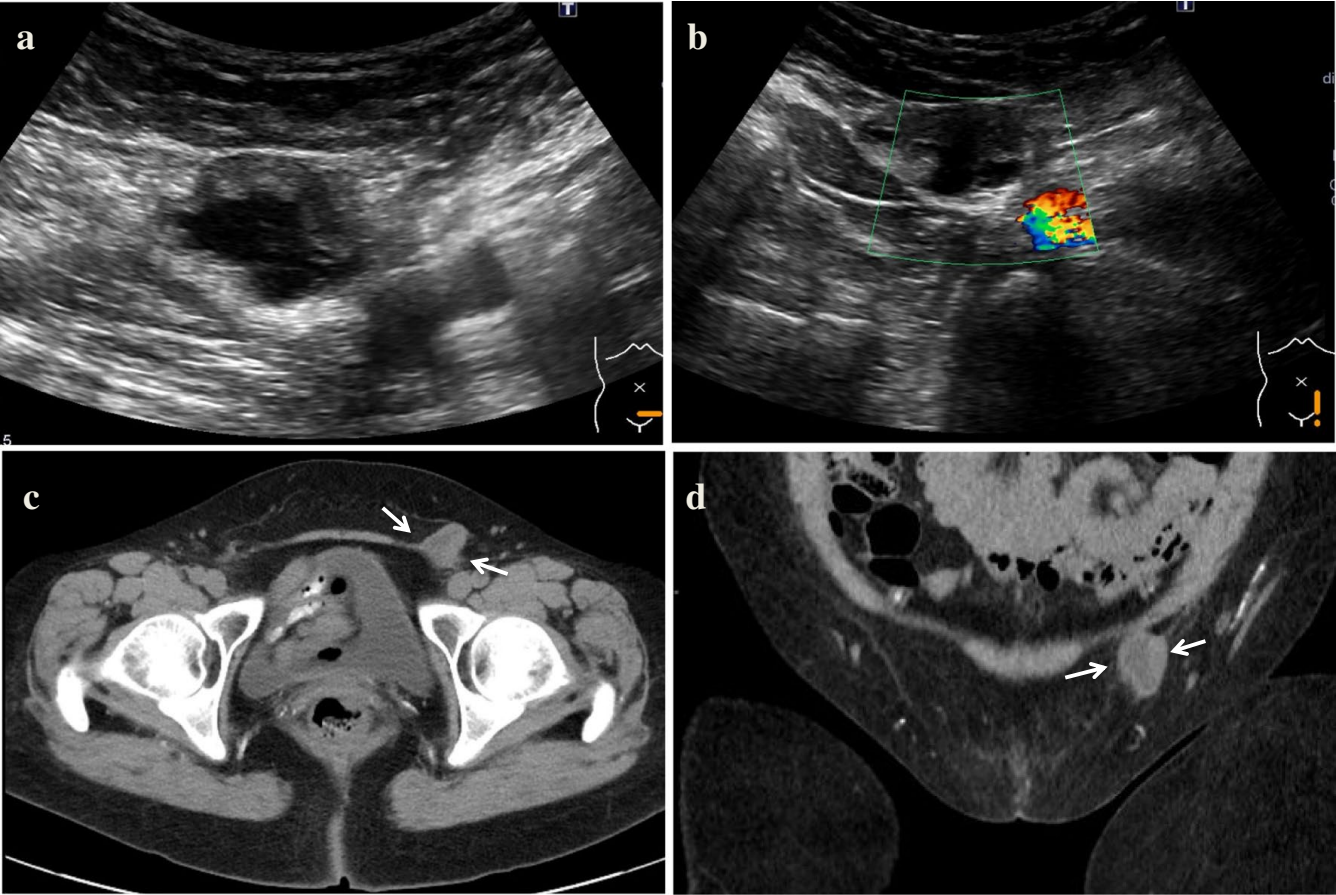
Ultrasound images a US showed a cystic lesion measuring 40 mm in diameter, which had unclear boundaries and appeared hypoechoic in the inguinal canal. b The color Doppler scan did not show any internal blood vessel distribution. c CT showed the inside of the cystic lesion was liquid, with irregular hypertrophy of the cyst wall. d CT coronal image shows that the cystic is not communicating with the abdominal cavity
